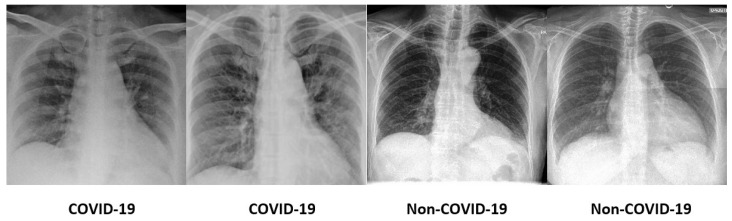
Some sample COVID-19 and non-COVID-19 images from the COVID-Xray-5k dataset.
